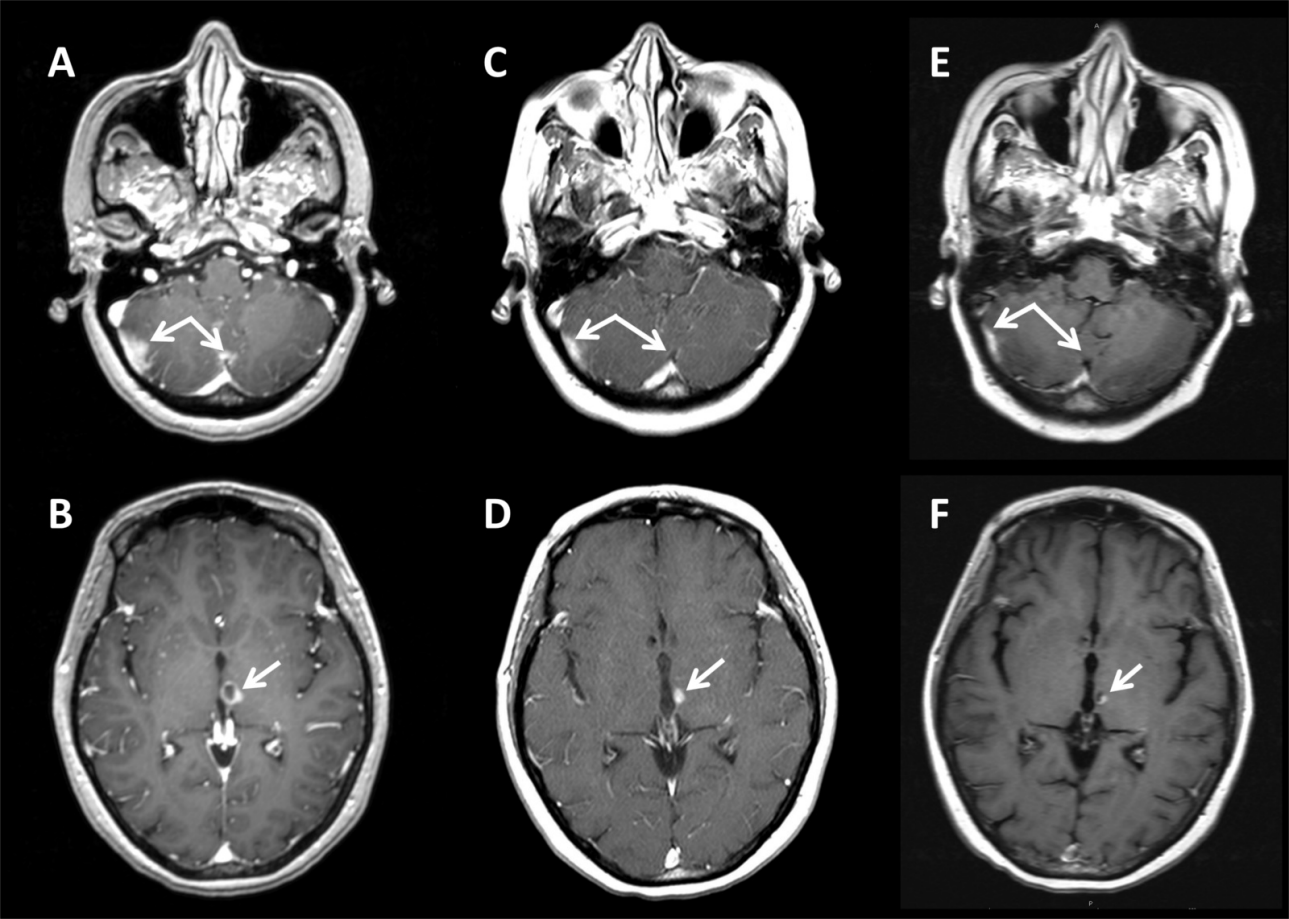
Brain MRI Findings Axial T1 post-contrast MRI shows areas of concern and response to treatment at two representative slices at the time of diagnosis of leptomeningeal relapse (A and B), one month post-radiosurgery (C and D), and five months post-radiosurgery (E and F). Dual arrows (A, C, E) highlight an area of bulky cerebellar disease and leptomeningeal involvement. Single arrows (B, D, F) highlight an area of bulky subventricular disease.
